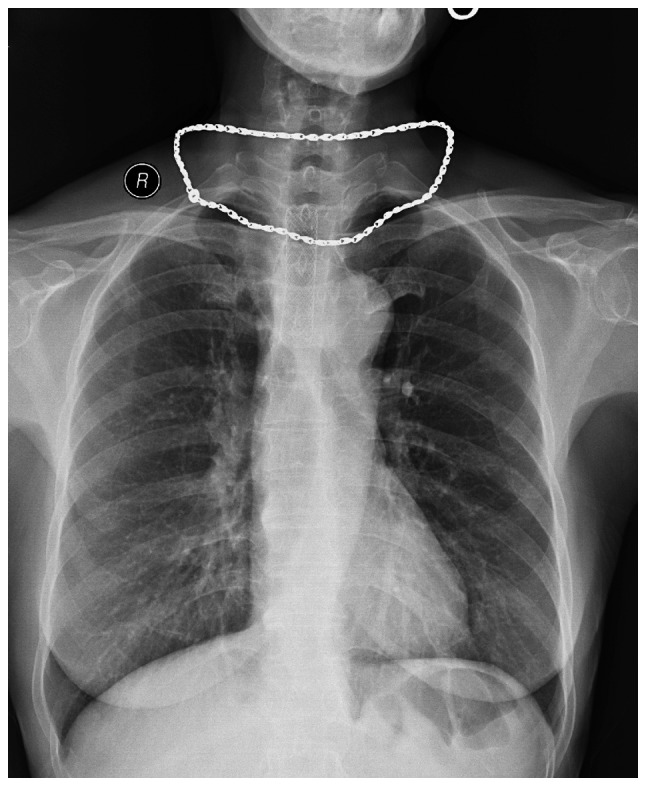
Chest radiography one day after surgery. Images revealed that the positioning and expansion of the metallic stent were successful.
